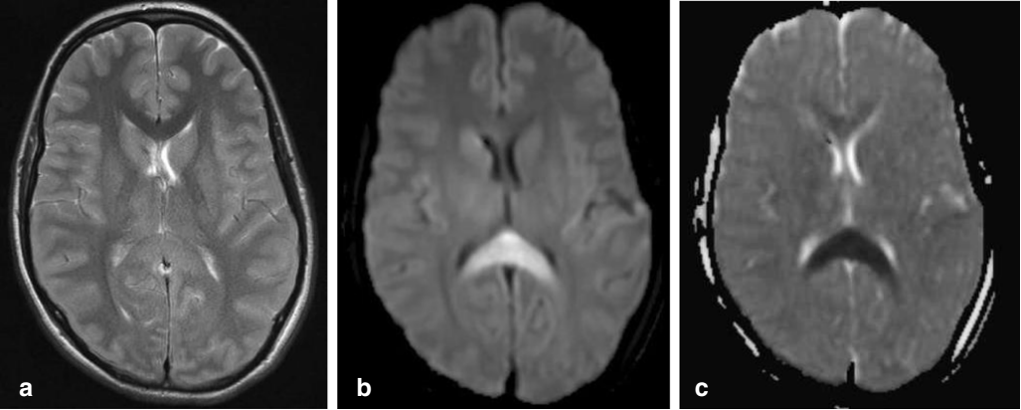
Fifteen-year-old girl post high energy  trauma. CT on day of trauma (not shown) showed no abnormalities. Axial T2 MRI (a) at day 6, post trauma, shows involvement of the entire splenium, with restricted diffusion on DWI (b) and ADC map (c). At 6-week follow-up, the patient had severe residual cognitive disturbance
